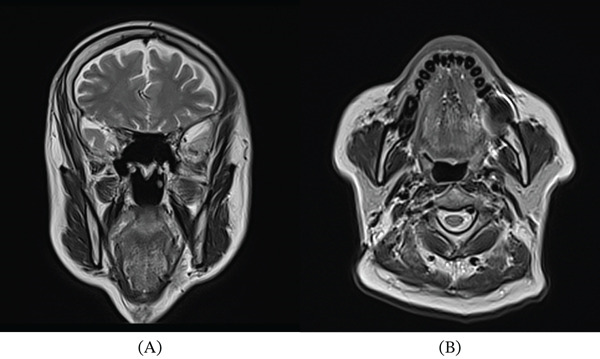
S/c MRI of the T2 face (January 12, 2025). (A) Coronal and (B) axial section.
